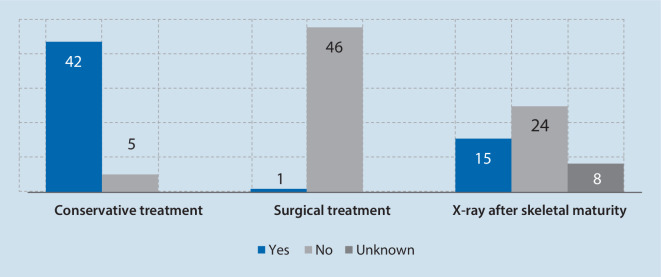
Selected treatment in patients with positive findings in neonatal hip sonography (Graf > 1) as well as rate of radiographic follow-up in patients with positive findings in neonatal hip sonography
